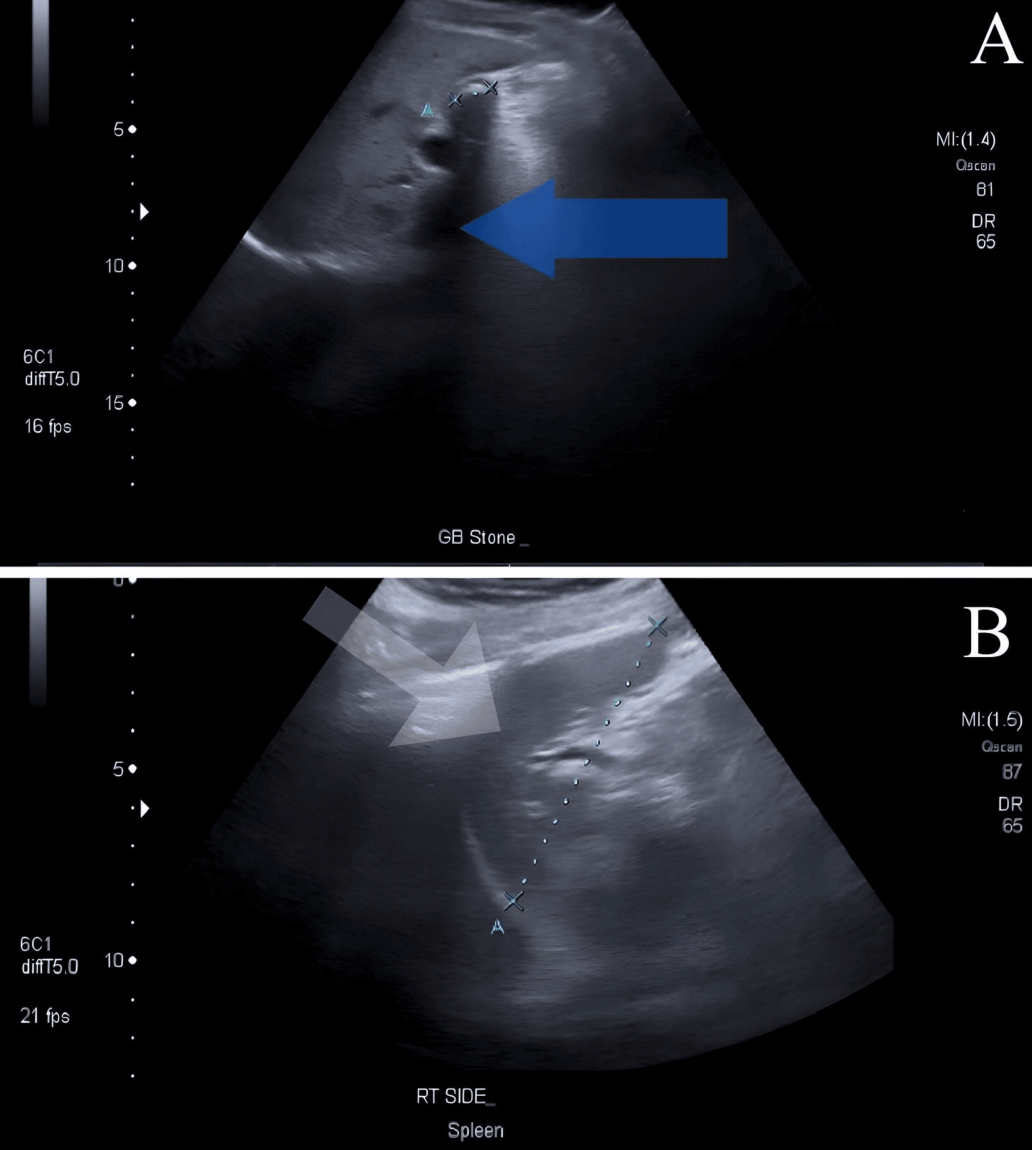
Abdomen US Multiple gallbladder stones with stone impacted in the neck (A) and posterior shadow (blue arrow). The spleen (white arrow) shows homogeneous and uniform parenchymal echogenicity and it is positioned on the right side (B). US: Ultrasound
